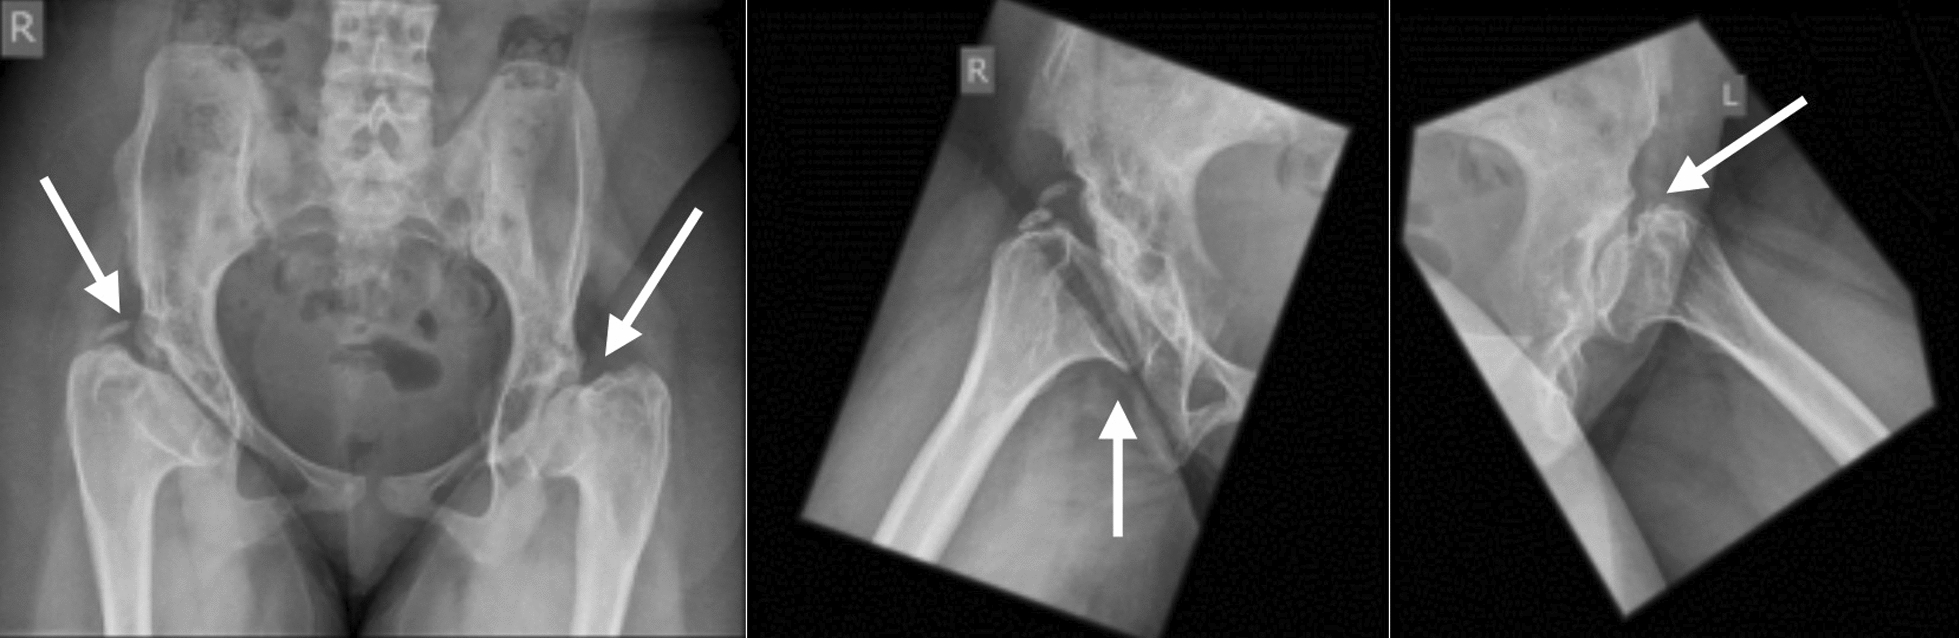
Pelvic X-ray showing marked flattening and resorption of both femoral heads with shallow acetabula, findings consistent with long-standing developmental dysplasia of the hip or avascular necrosis
